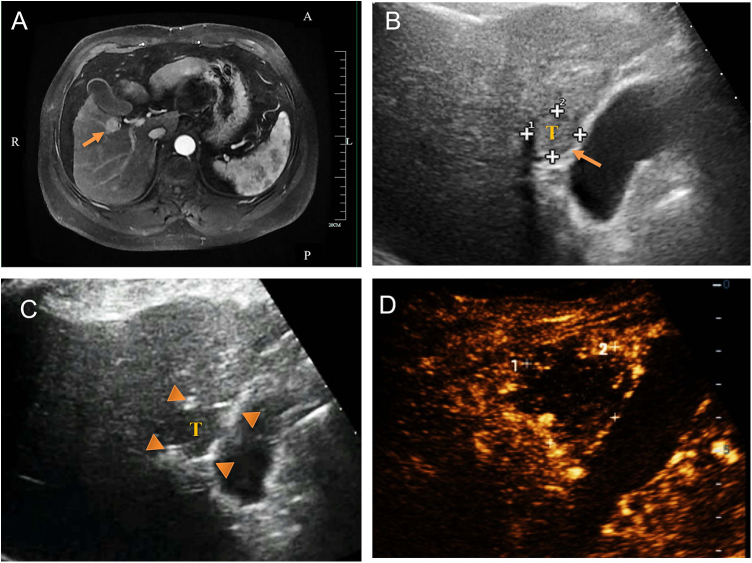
Ultrasound-guided nanosecond pulsed electric field (nsPEF) procedure for HCC adjacent to gallbladder. (A) Pre-ablation contrast-enhanced MRI revealed a hepatocellular carcinoma adjacent to gallbladder (arrow). (B) Ultrasound showed a hypoecho tumor located in close contact with gallbladder (arrow). (C) Two 19-gauge unipolar nsPEF electrodes (triangle) were inserted parallelly and percutaneously around the tumor. (D) The contrast-enhanced ultrasound image revealed no enhancement after ablation.
